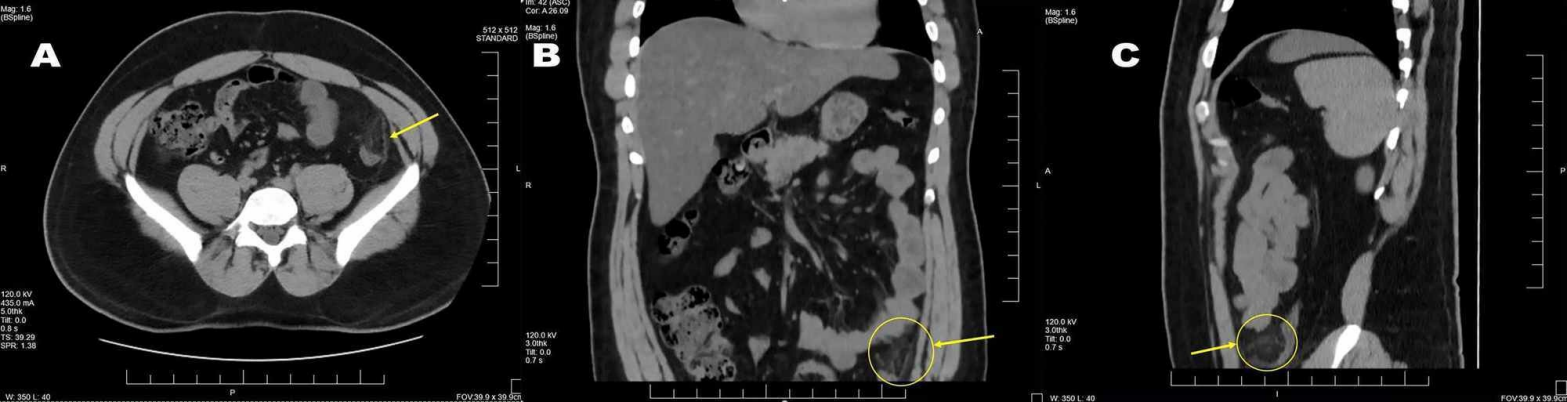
(A) Axial, (B) coronal, and (C) sagittal non-contrast CT images showing a fat-containing nodule adjacent to the descending colon with surrounding inflammatory stranding (arrows).
